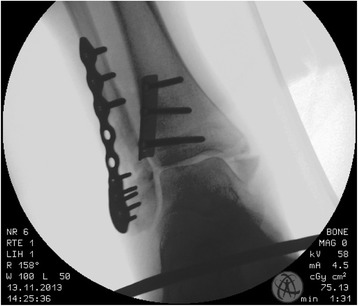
Intraoperative X-ray; Mortise view after fixation of the posterior malleolus with a with an three-hole antiglide-plate and a locking plate for the fracture of the lateral malleolus
